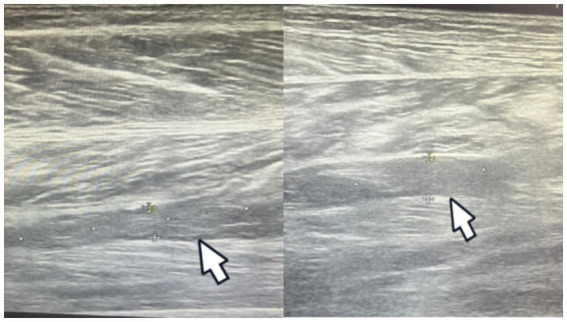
Venous ultrasound of the legs on admission showing thrombosis of the upper segment of the left tibial posterior vein (Left image) and bilateral intermuscular veins, with maximum dimensions of 5.2 × 0.6 cm on the left side and 6.2 × 0.9 cm on the right side.
